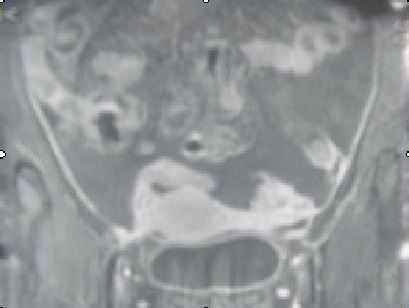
Pelvic MRI T2, image located in the left side of the uterus measuring 08 cm with dual component solid and cystic
